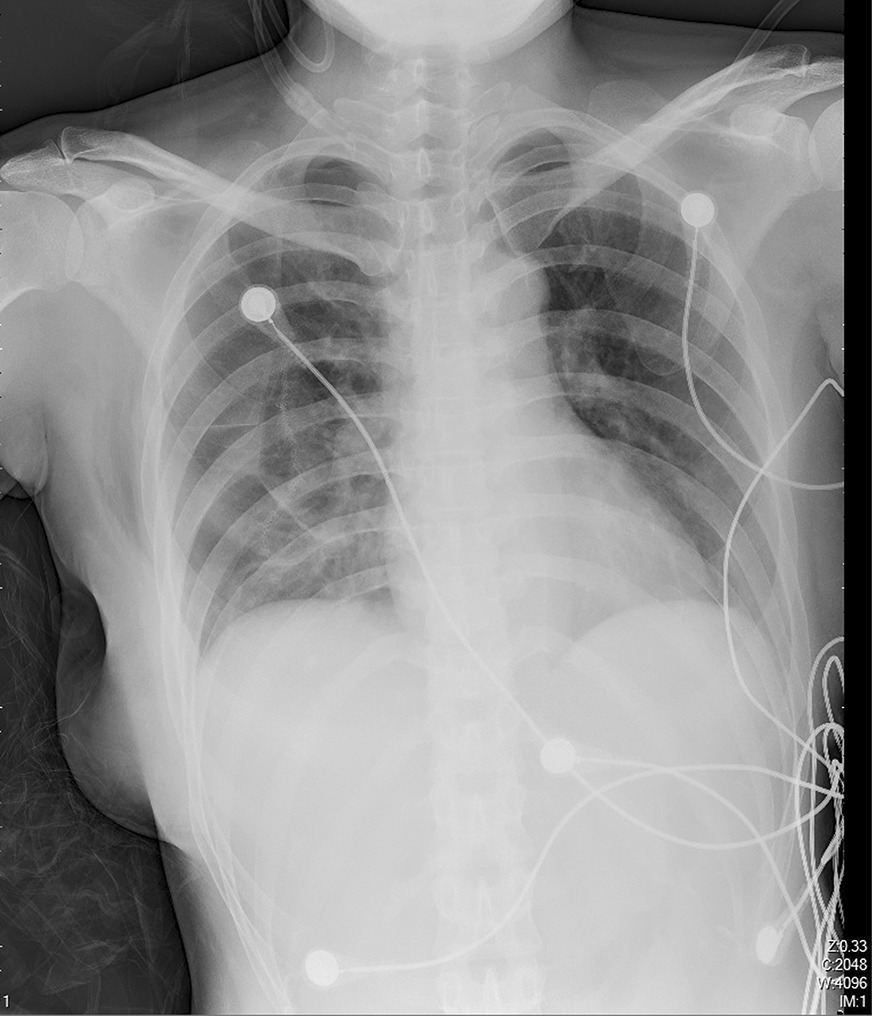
Chest radiography was performed post-operatively
